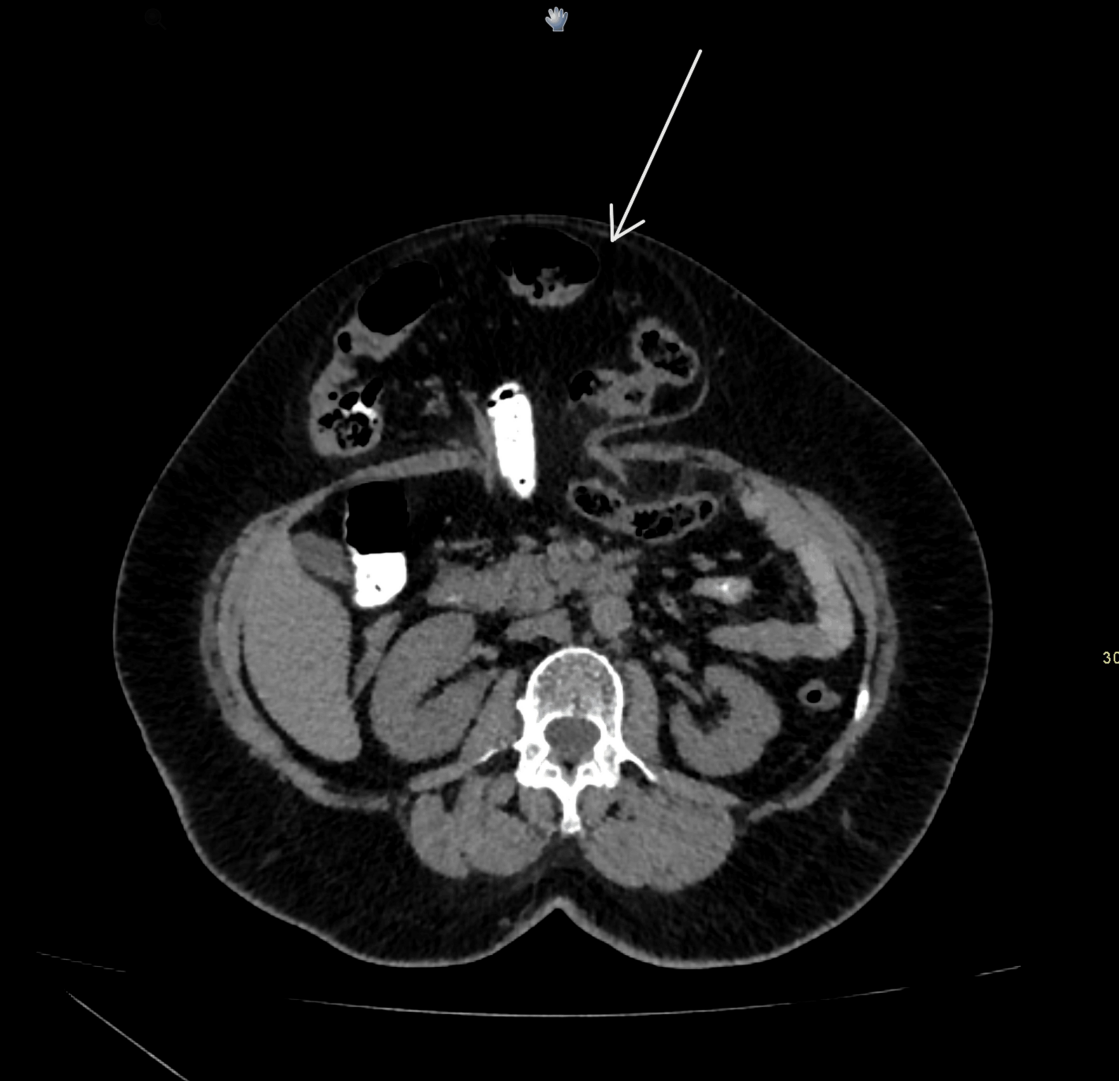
Non-contrast CT with oral contrast showing an axial view with bowel in the hernial sac (arrow)
